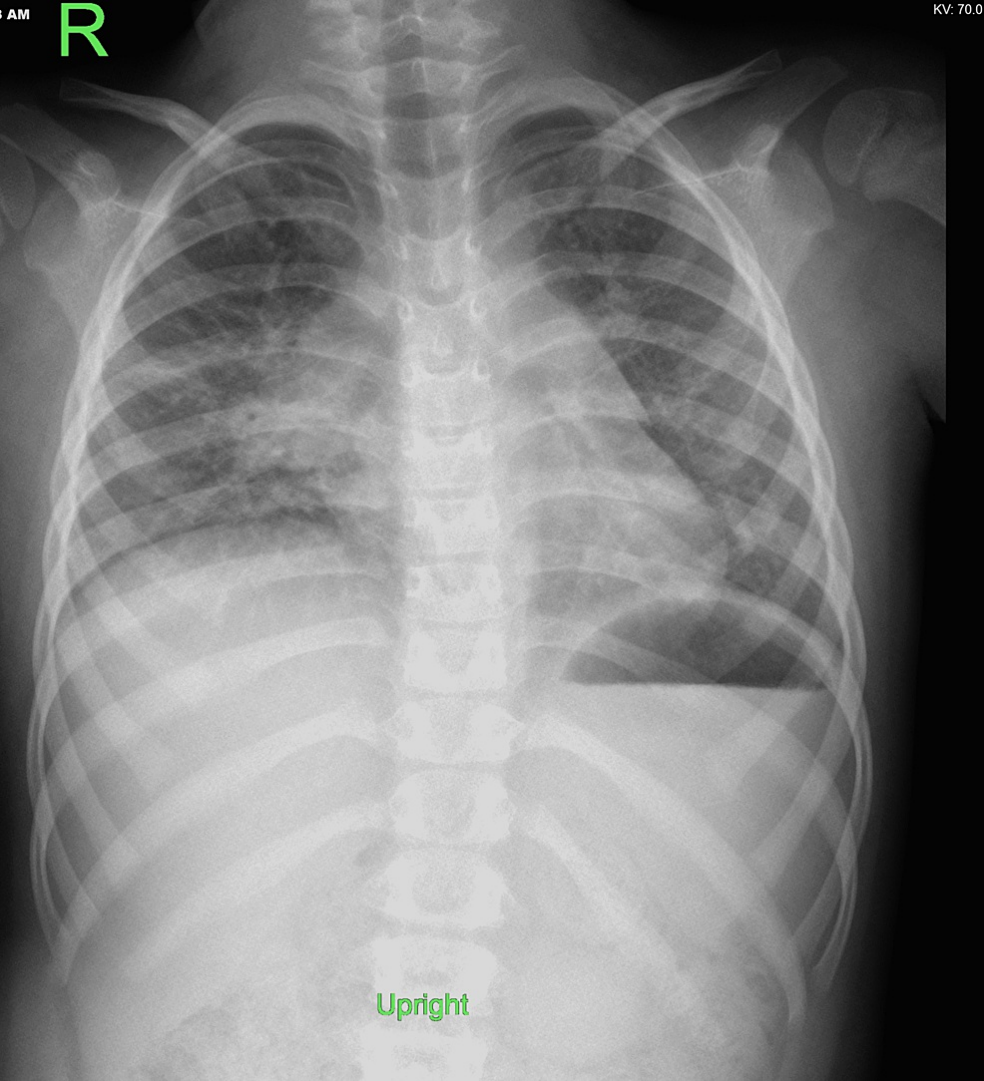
Chest X-ray on admission showed right lung midzone and left retrocardiac lower and mid-zone air space opacity with air bronchograms.
